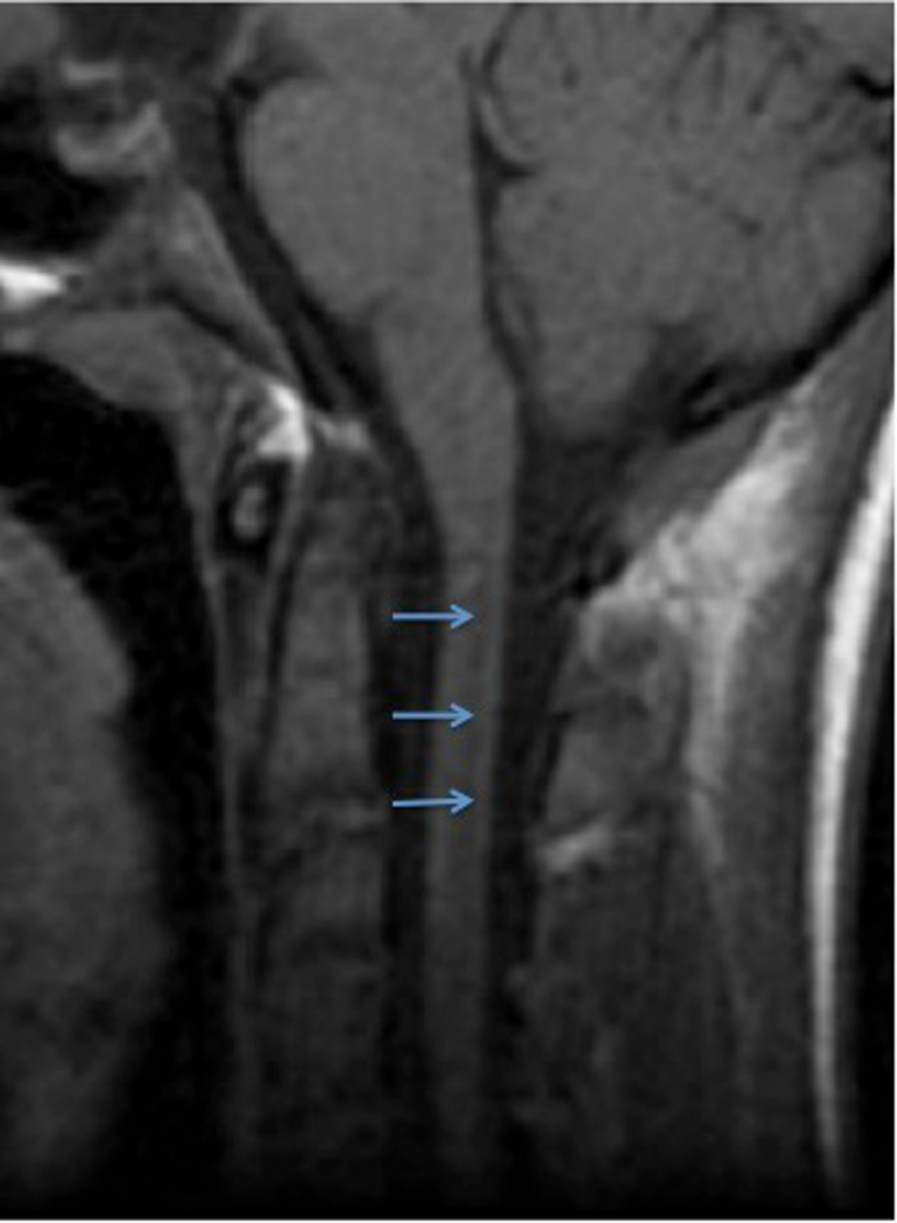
T1-weighted sagittal MRI noting a normal central canal (arrows).
